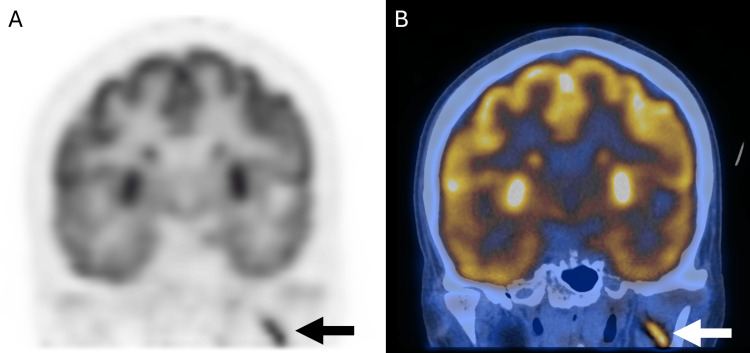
PET-only (A) and PET/CT fusion (B) coronal images showing linear hypermetabolism along the mandibular branch (V3) of the left trigeminal nerve
